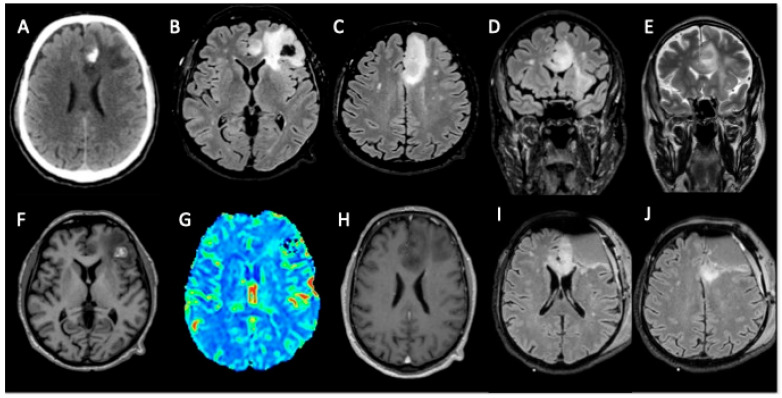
Polymorphous low-grade neuroepithelial tumor of the young (PLNTY) in a 62-year-old man with epileptic seizure scan: (A) MRI FLAIR (B–D,I,J), T1WI (F), T2WI (E), post-contrast T1-weighted images (H), DSC perfusion (G). A cortico-subcortical infiltrative tumor is present in the left frontal region, involving the corpus callosum through which it extends to the right cingulate gyrus. Perilesional edema, calcifications, and hemorrhagic components are evident. No enhancement is observed after contrast administration. The lesion does not show any significant increase in perfusion (rCBV). The postoperative MRI study (I,J) shows residual tumor in the genu of the corpus callosum and in the left frontomesial cortico-subcortical region.
